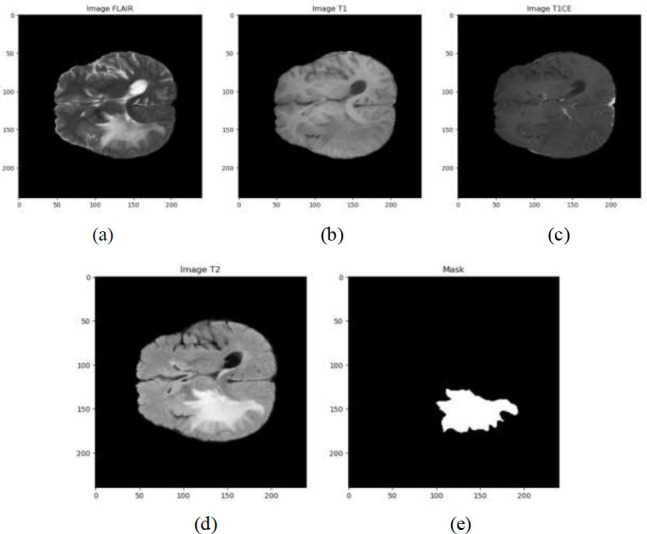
Visualization of preprocessing image (a) FLAIR image (b) Image T1 (c) Image T1CE (d) Image T2 (e) Mask.
